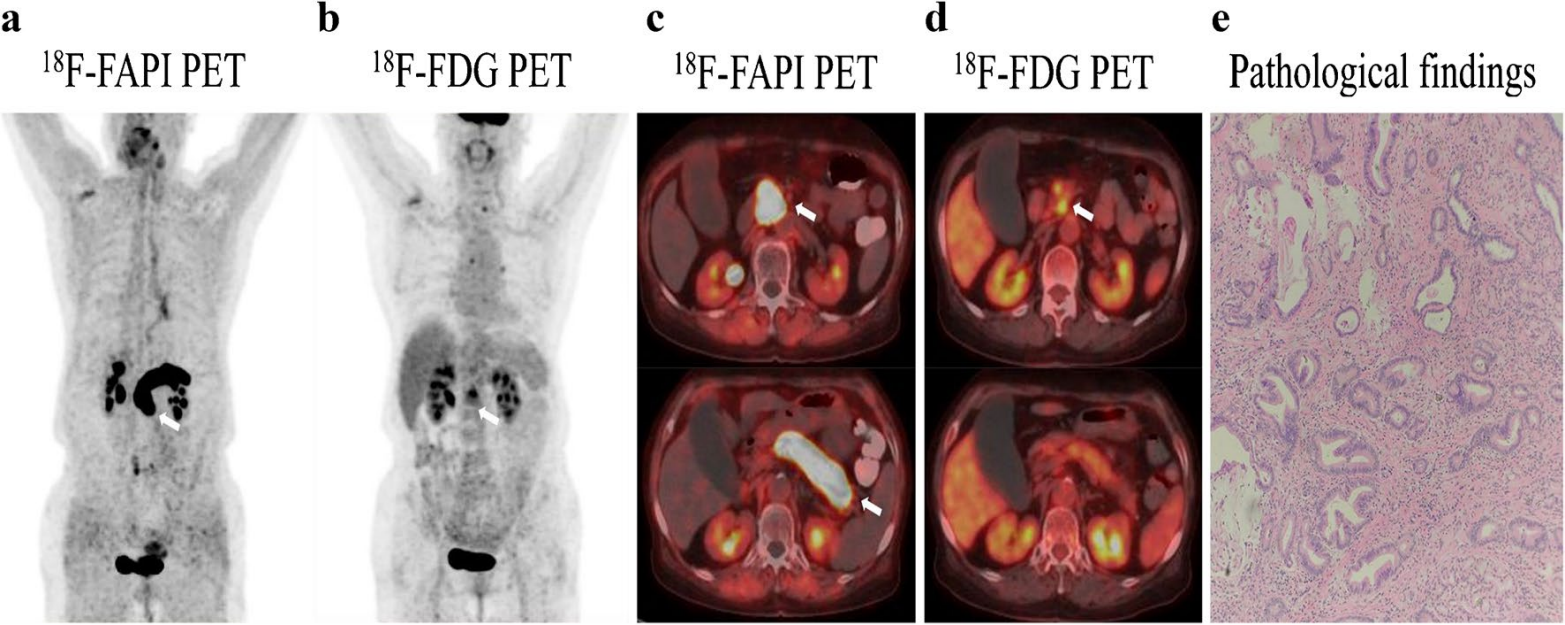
a, c Images from ^18^F-FAPI-04 PET/CT show an irregular lesion in the pancreas with intense metabolic activity, which strongly suggests pancreatic cancer. Intense and diffuse ^18^F-FAPI-04 uptake (SUVmax = 9.3) in the primary tumor and the body and tail of the pancreas was found. b, d Images from ^18^F-FDG PET/CT show two ill-defined nodules in the pancreas with low metabolic activity (SUVmax = 9.3). e The pathological findings derived from surgical resection are indicative of pancreatic cancer
